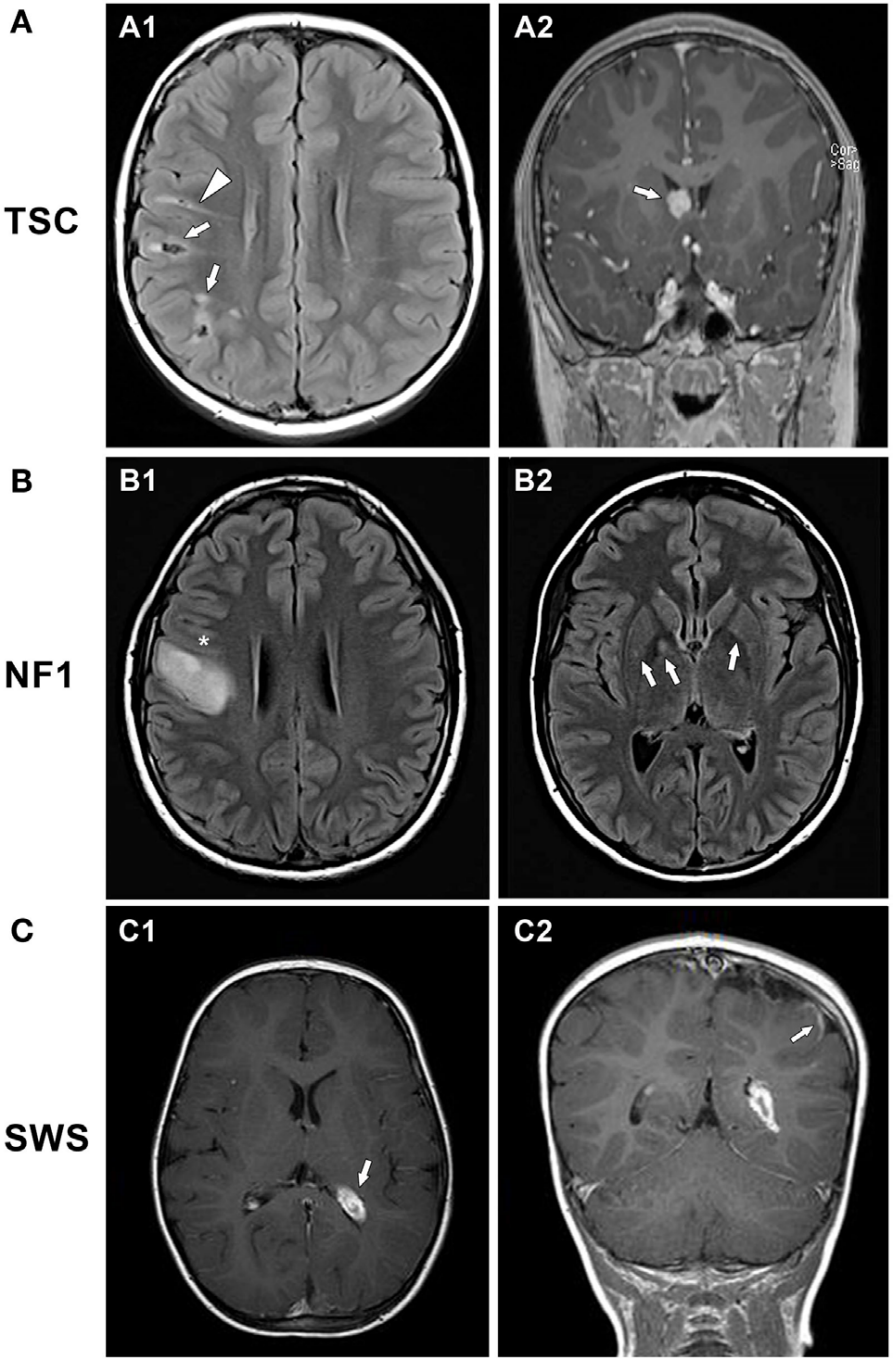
Examples of brain MRI scans in patients with neurocutaneous disorders. (A) 8-year-old boy with tuberous sclerosis complex. (A1) Axial fluid attenuation inversion recovery (FLAIR) and (A2) coronal contrast-enhanced T1-weighted images show multiple cortical/subcortical, partially calcified mixed FLAIR-hyper/hypointense tubers in the right front parietal lobes (arrows, A1), FLAIR-hyperintense migration lines (triangle, A1), and a homogeneously enhancing, round subependymal giant cell astrocytoma within the right foramen of Monro (arrow, A2). (B) 16-year-old girl with neurofibromatosis type 1. (B1) Axial FLAIR and T1 post-contrast images showing a large expansile lesion centered at the right precentral gyrus (*) indicating a low-grade glioma. (B2) Axial T2 FLAIR image reveals focal areas of signal intensity (arrows) in the right globus pallidus and putamen and similar but to a lesser extent on the contralateral side, representing nonspecific unidentified bright objects. (C) 21-month-old boy with Sturge–Weber syndrome. (C1) Axial T1-weighted post-contrast image showing left hemisphere atrophy with prominent choroid plexus glomus (arrow). (C2) Coronal T1-weighted post-contrast image with occipital-parietal leptomeningeal enhancement (arrow).
